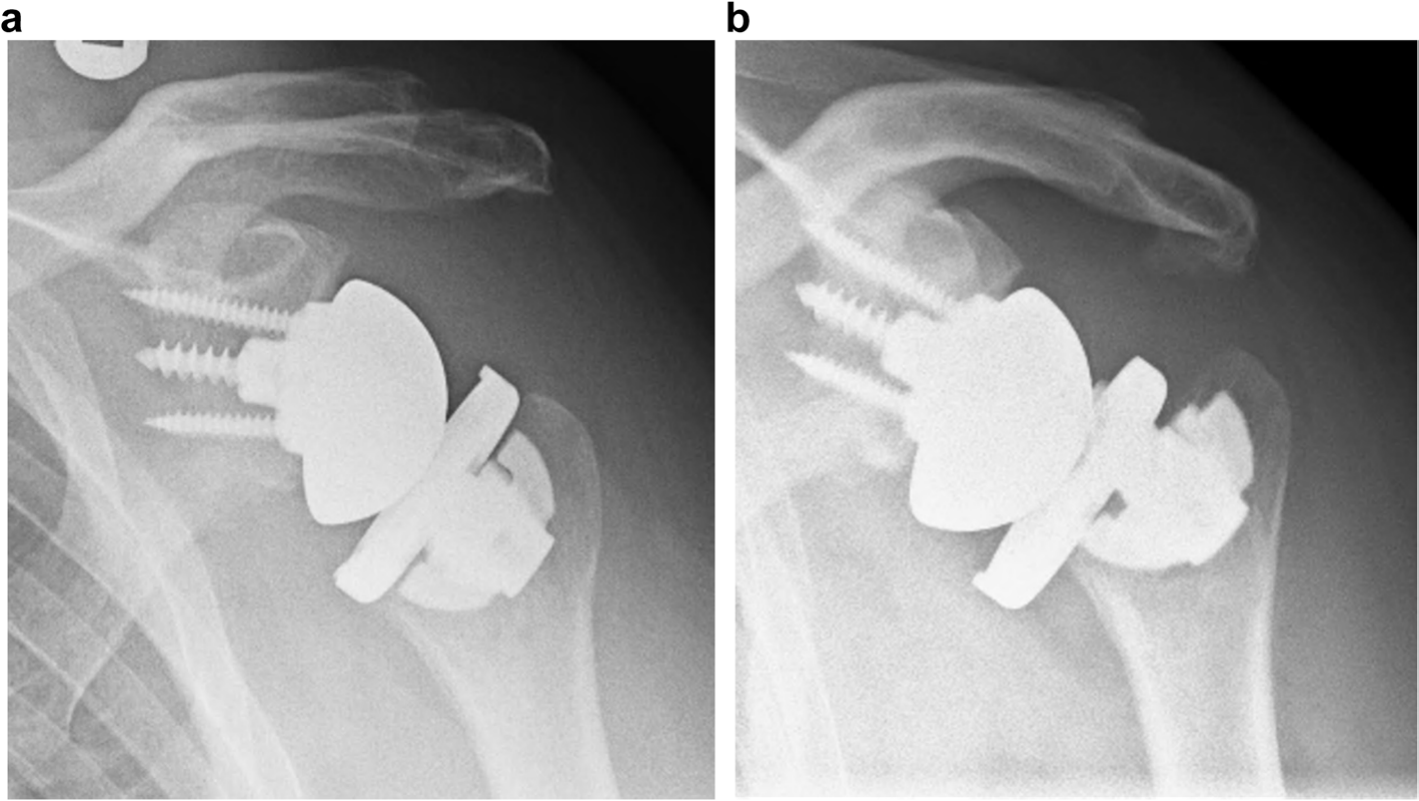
X-ray imaging of a patient with implant failure. Postoperative imaging (a) and breakage of the central peg of the humeral tray with disengagement from the Nano anchor 3 years after primary implantation (b)
